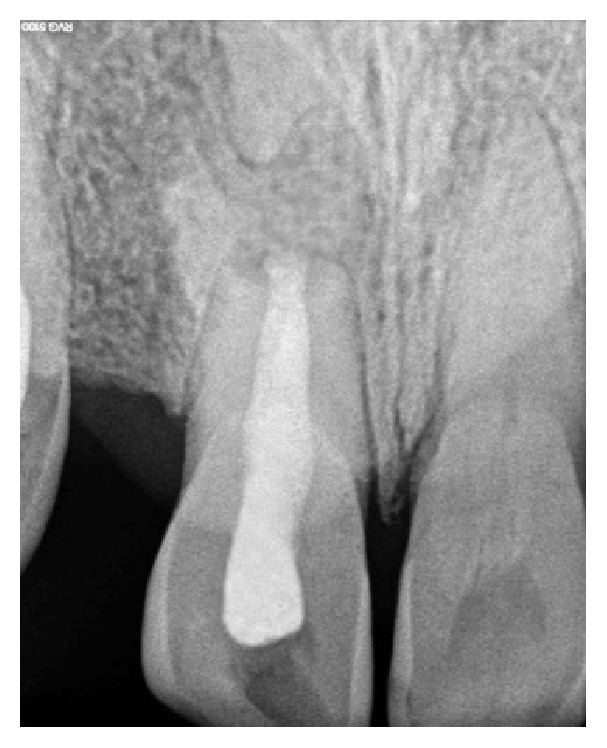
10-month follow-up of the case shows completely resorbed matrix and bone healing in the periapical area.
